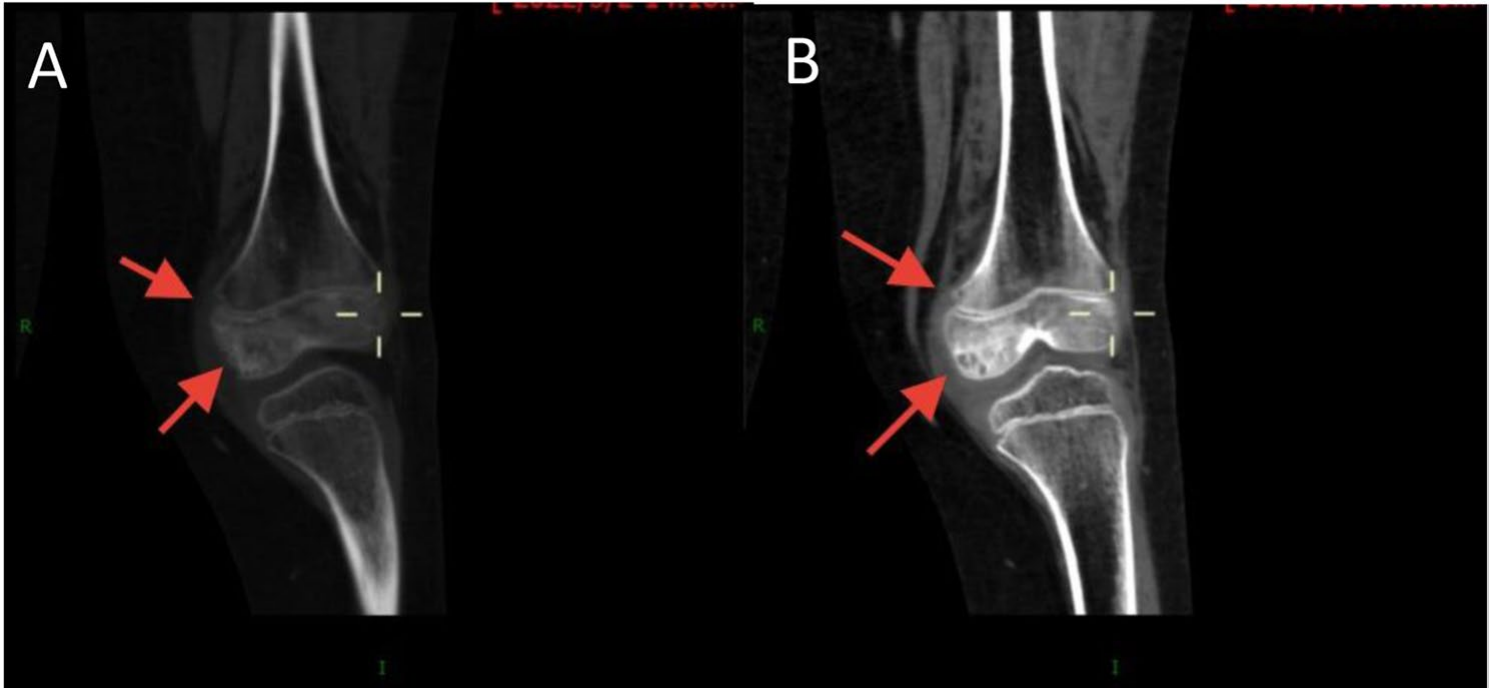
Permeative/moth-eaten infiltration pattern (Pattern 1) of KHE in an 8-year-old male with an abnormal gait. (A) Coronal CT image (bone window) of the right proximal femur demonstrates multiple small, dotted, and moth-eaten osteolytic lesions (white arrows) with ill-defined margins, involving both the epiphysis and metaphysis. (B) Sagittal CT reconstruction shows a prominent tubular or channel-like lucency (black arrow) traversing the metaphysis, a characteristic finding in this pattern. Note the absence of periosteal reaction or a soft-tissue mass. (C) Coronal T2-weighted MRI shows heterogeneous hyperintensity throughout the affected epiphysis and metaphysis
